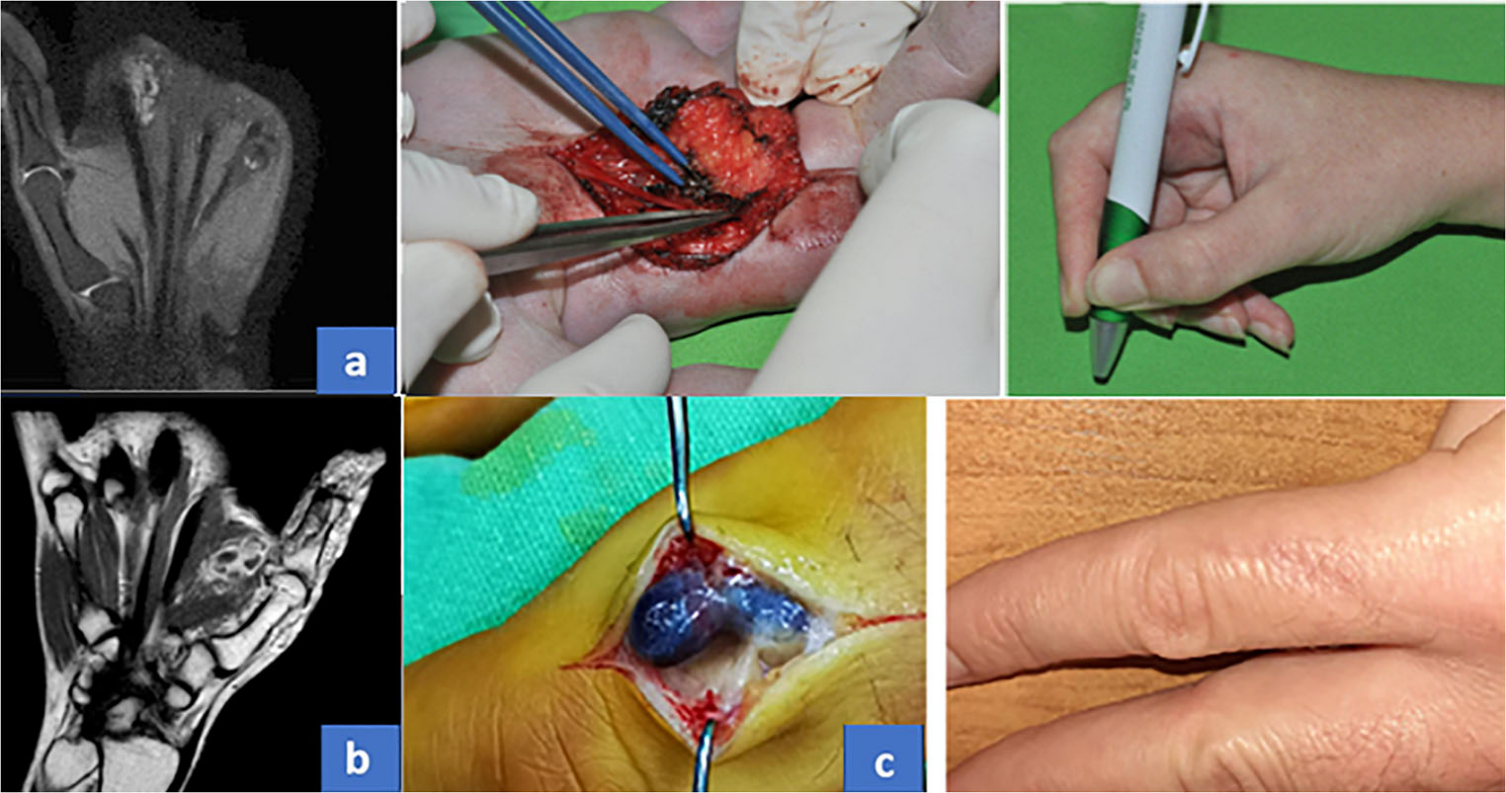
a From left to right: T2-weighted anterio-posterior MRI image of Patient No 1., Surgical excision, Post-operative function. b T2-weight AP MRI of Patient No 3. c Surgical excision performed for Patient No 6., result at 6-months follow-up
